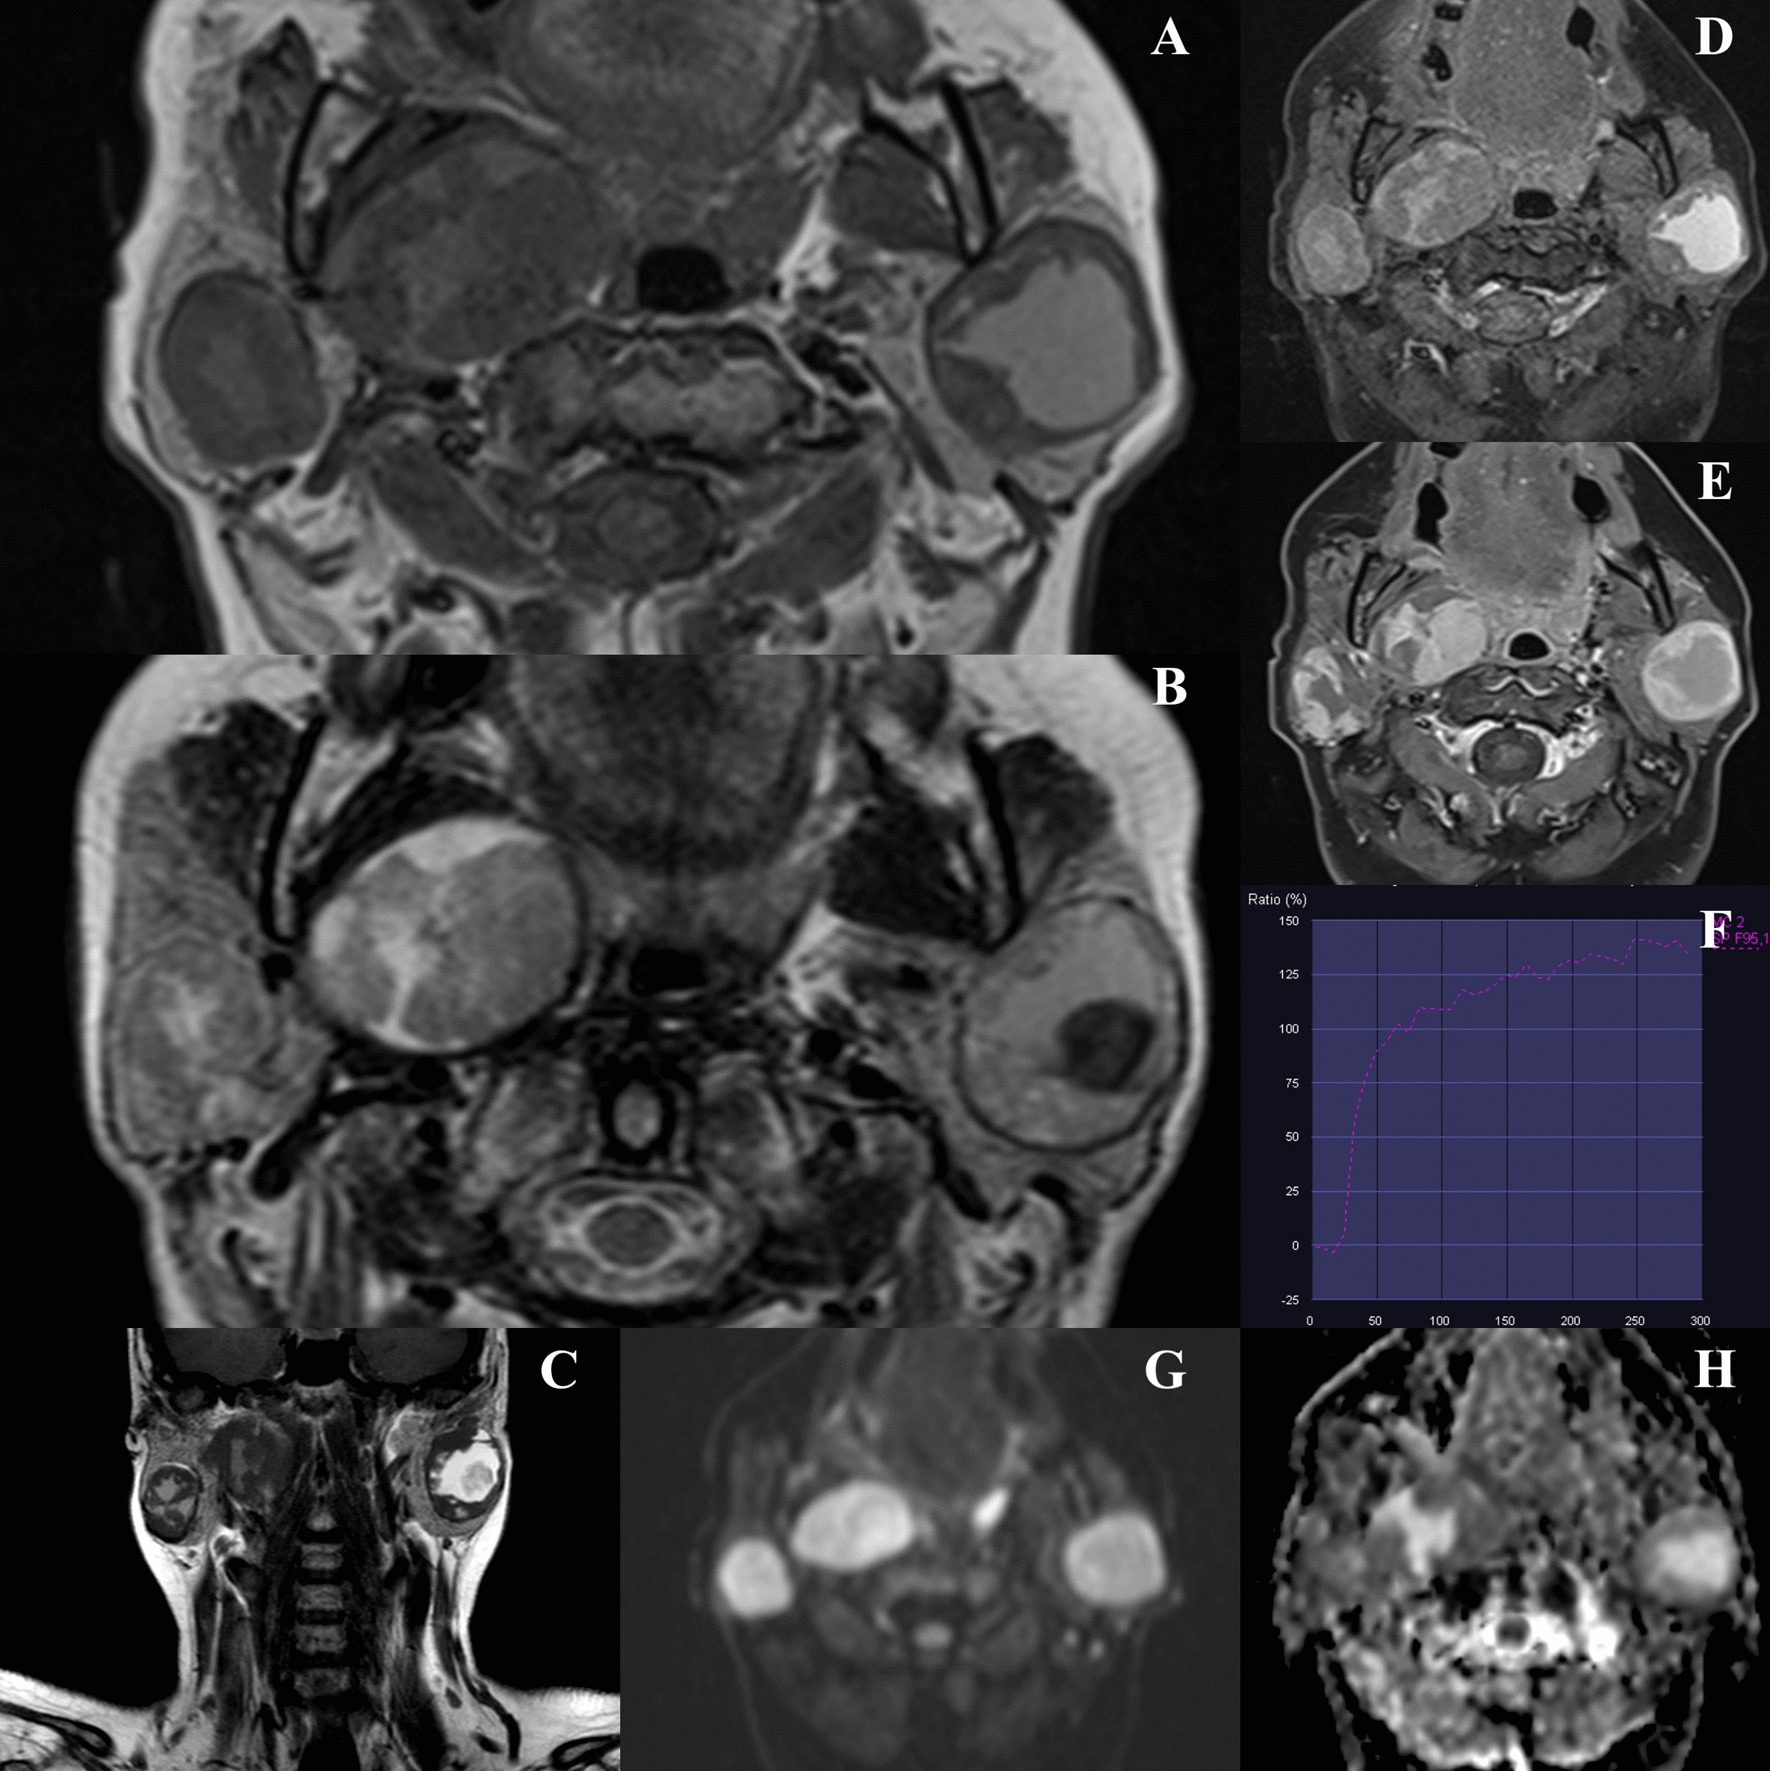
MRI of bilateral multifocal parotid basal-cell adenomas. The examination shows heterogeneous lesions, with central cystic areas, partially with higher protein content and signs of bleeding, and peripheral solid components (A. T1-weighted axial image, B. T2-weighted axial image, C. T2-weighted coronal image and D. T2-weighted fat-saturated axial image). Solid components of the tumours present vivid enhancement post-gadolinium-based contrast agent (E. contrast-enhanced T1-weighted fat-saturated axial image) and the time-intensity curves obtained on the basis of dynamic contrast-enhanced sequence show a continuous uptake of the contrast agent suggestive of adenomas (F). Tumors did not show signs of diffusion restriction with ADC values of approximately 1.48 × 10^−3^ mm.^2^/s (G. diffusion-weighted axial image, b 1000, H. ADC map)
